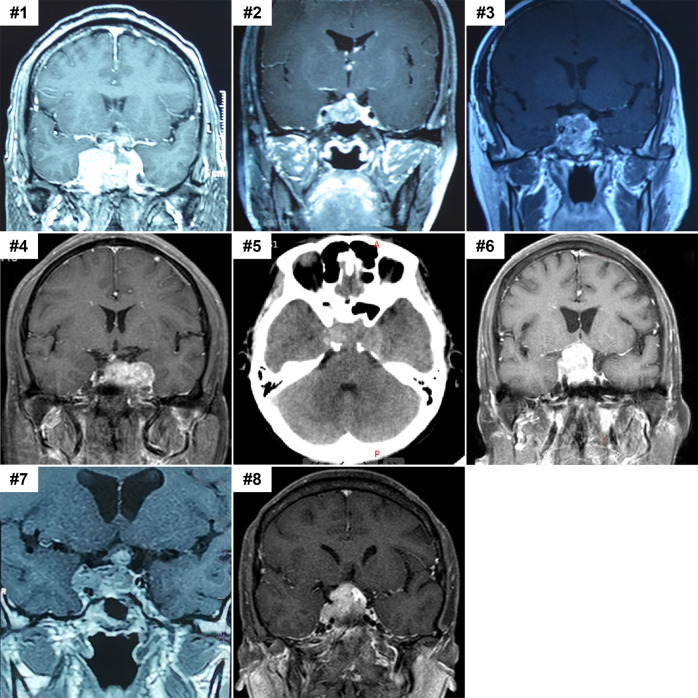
Pre-operative imaging features of the eight primary adult sellar SMARCB1/INI1-deficient tumors. Images shown are pre-operative magnetic resonance images (cases #1–4 and #6–8) and CT image (case #5). All tumors were lobulated or lumpy solid masses located in the sellar region involving intrasella, suprasella or parasella. After enhancement, the lesions displayed homogeneous or heterogeneous contrast enhancement in cases #1–4 and #6–8, CT image showed slightly hyperdense signals in case #5.
